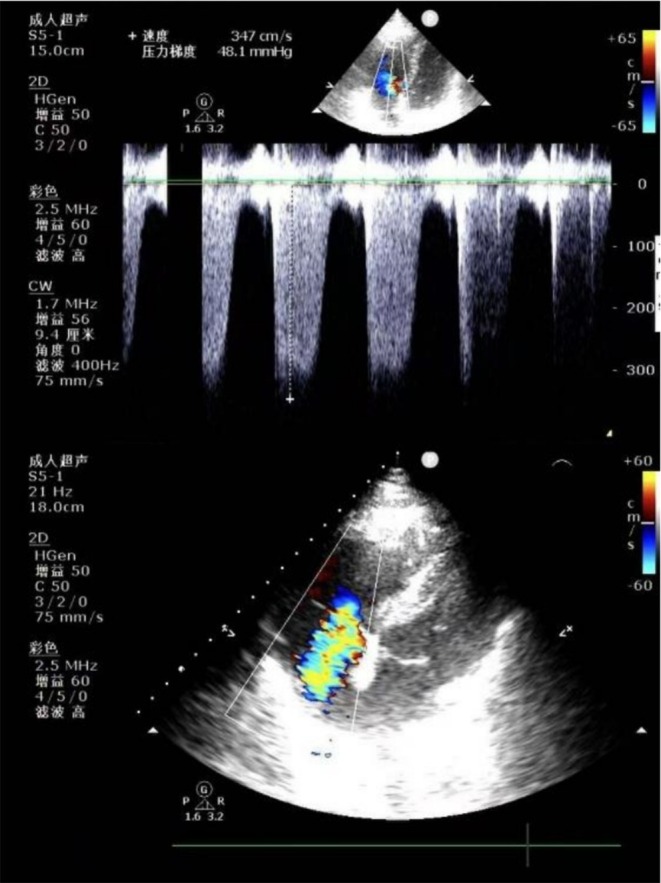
Preoperative and postoperative echocardiography.
